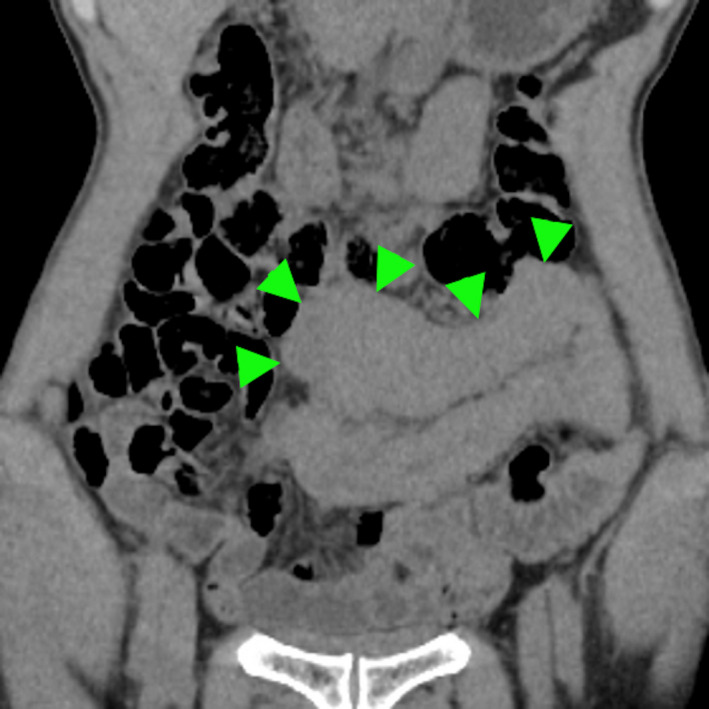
Abdominal computed tomography revealed small intestinal wall thickening (arrowheads)
